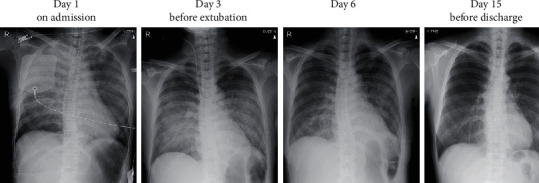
The course of chest X-ray images from admission to discharge. Images on admission (day 1) showed bilateral interstitial opacities, which gradually resolved during hospitalization.
